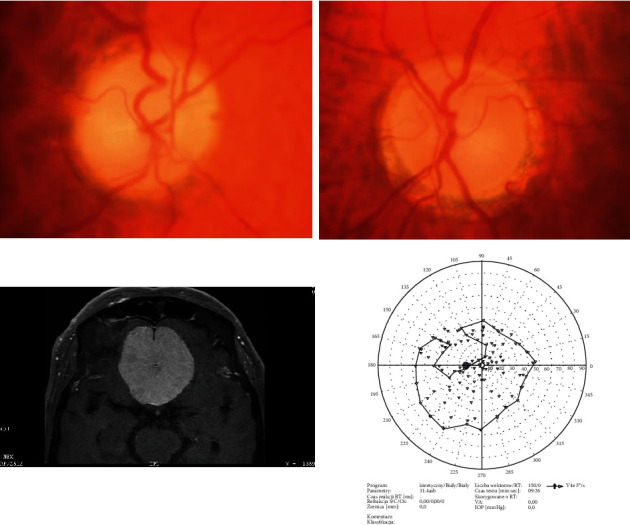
M/68 with positive history of glaucoma. The reason for neuroimaging was the decrease in BCVA from BE: RE from 0.8 to no light perception and LE from 0.8 to counting fingers up to 1.5 m with IOP at the level of 8-10 mmHg. MRI scans showed intracranial meningioma with the diameter 5.5 cm.
